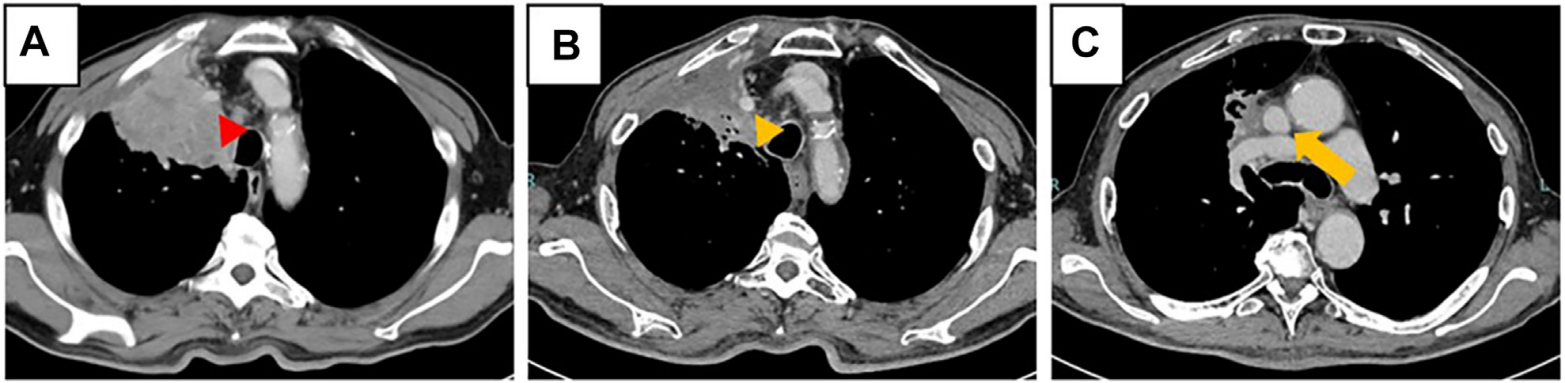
(A) Contrast-enhanced chest computed tomography before chemoradiotherapy, with the red arrowhead indicating the right brachiocephalic vein (right BCV). (B and C) Contrast-enhanced chest computed tomography after chemoradiotherapy, with (B) the orange arrowhead indicating the BCV and (C) the orange arrow indicating the superior vena cava. After chemoradiotherapy, the tumor size was reduced, and vascular invasion was mild.
